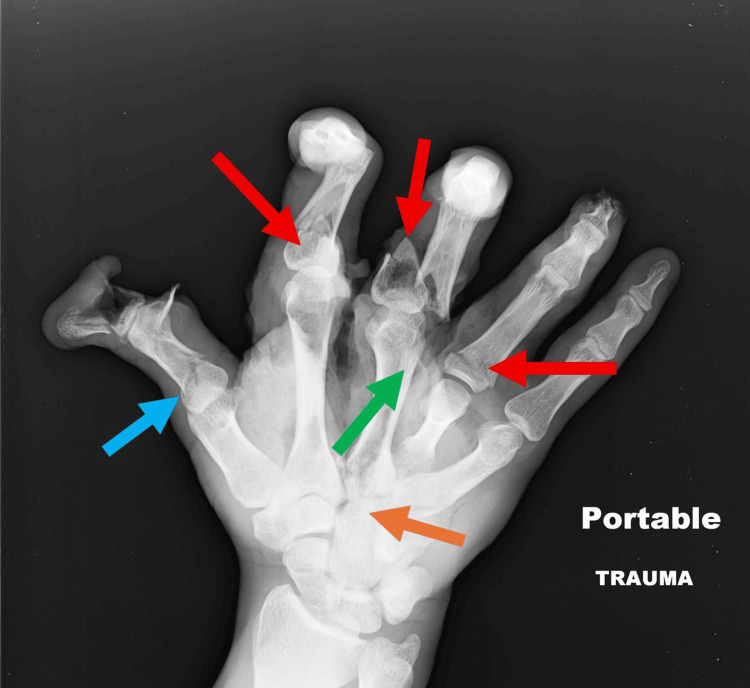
Preoperative radiograph of the right hand Preoperative anteroposterior view of the right hand demonstrating fractures at the base of the proximal phalanx of the index-ring fingers (red arrow), fracture of the proximal and distal phalanx of the thumb (blue arrow), fracture of the third metacarpal (green arrow), and dislocations of the third-fifth CMC joints (orange arrow). CMC, carpometacarpal
